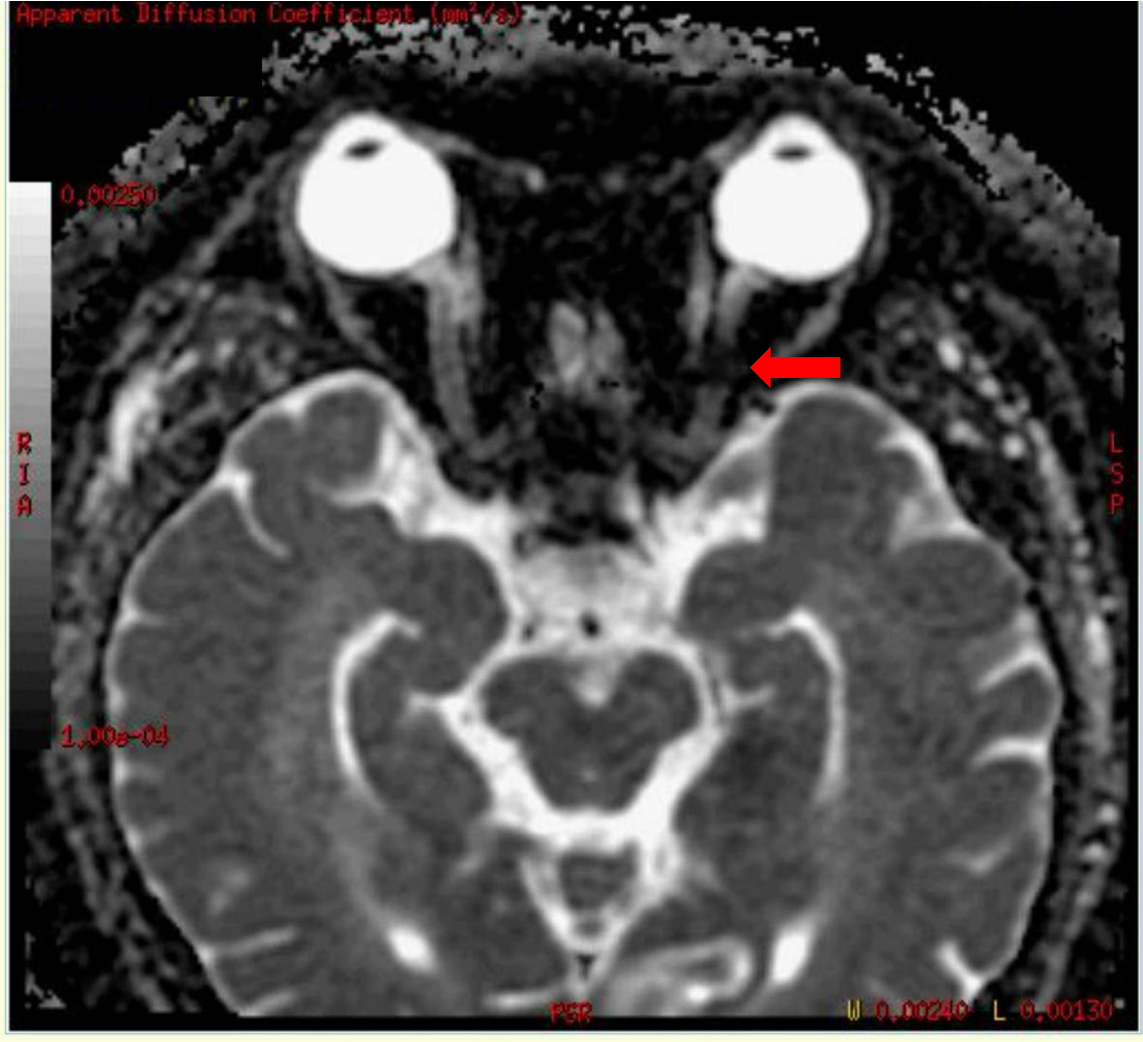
The hypointensity area shown on the ADC correlates to the hyperintensity area of DWI (Red arrow)
